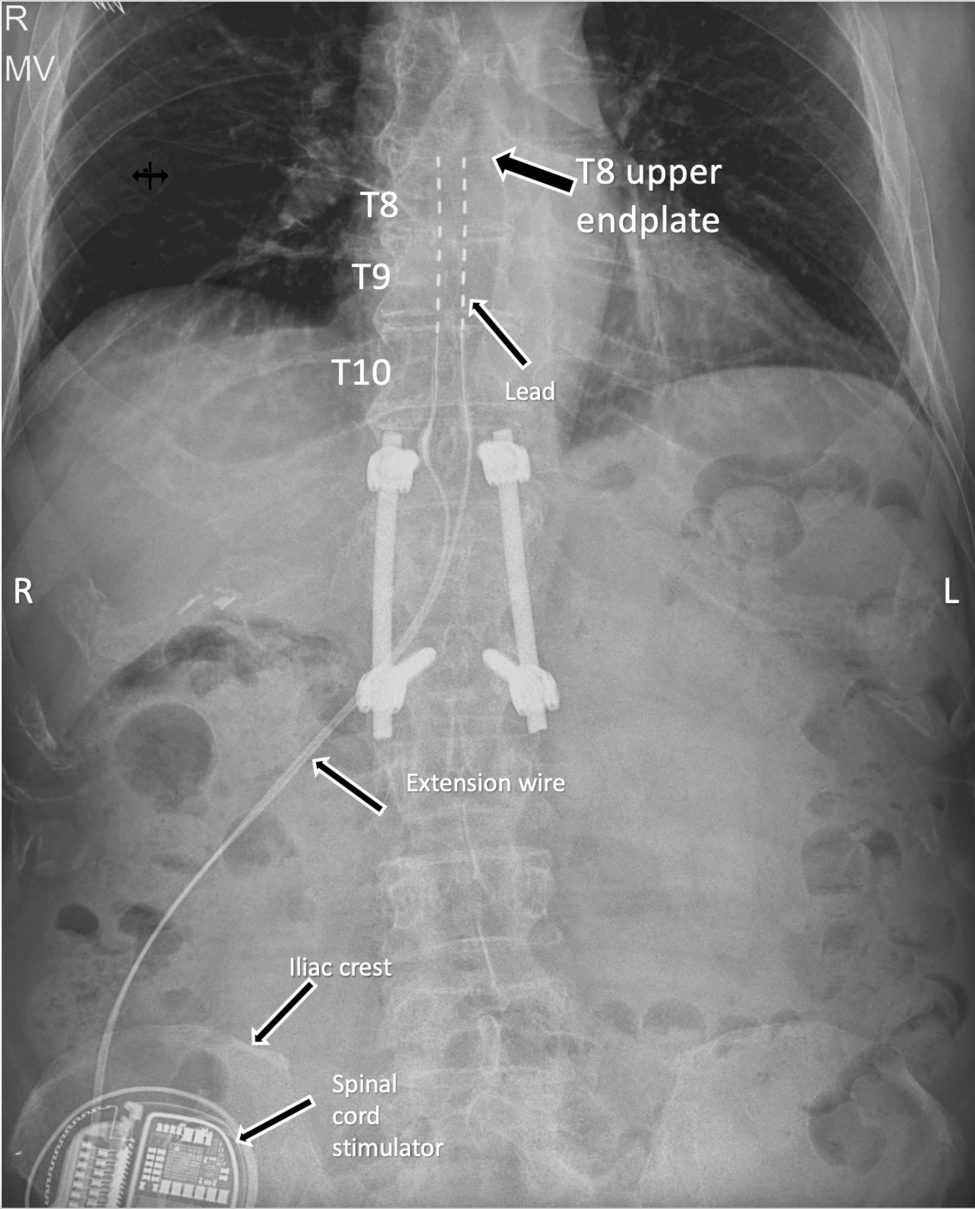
Anterior-posterior X-ray in admission to emergency room covering electrodes and programmer with no hardware migration, disconnection or lead fracture. Black arrows showing positioning of leads, extension wire, and spinal cord stimulator) T8: 8th thoracic vertebra; T9: 9th thoracic vertebra; T10: 10th thoracic vertebra; L: left; R: right
